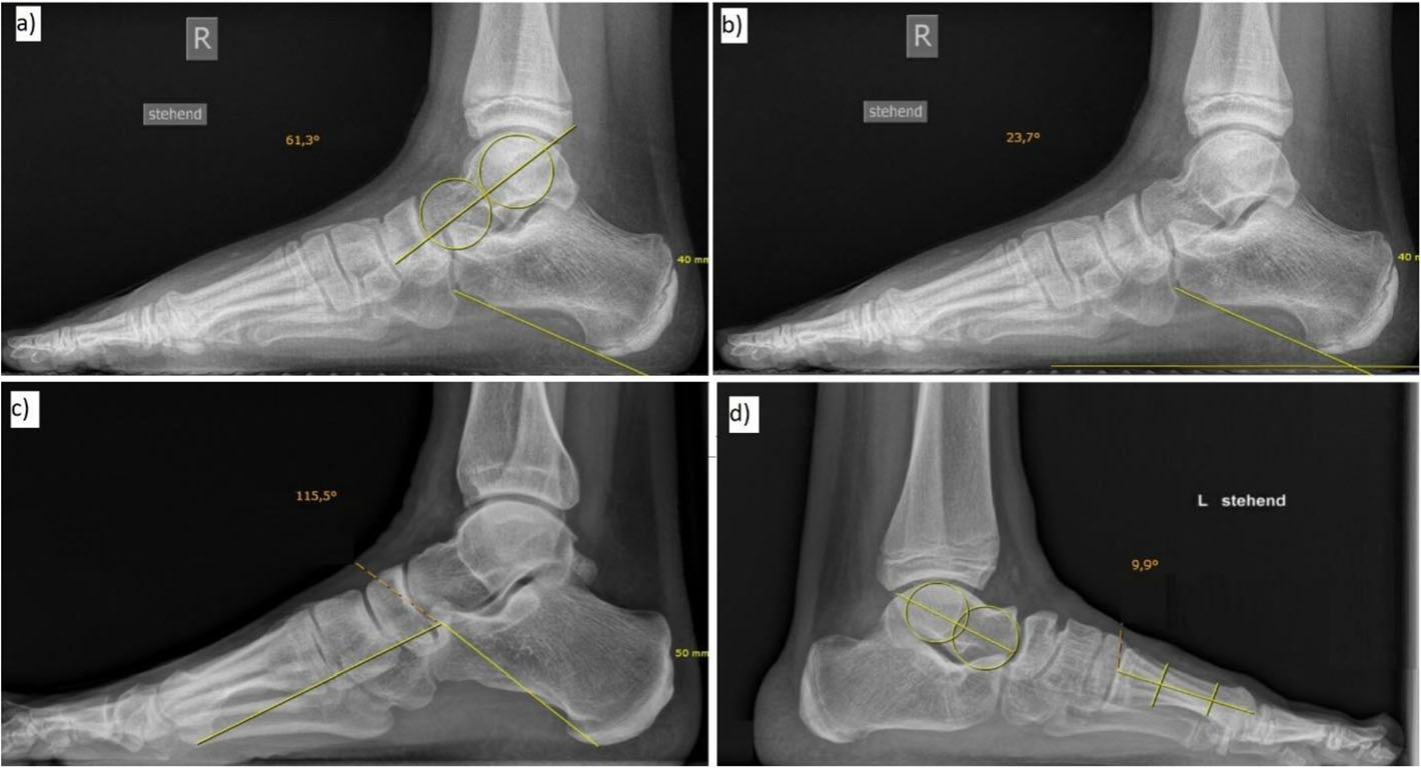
Radiographic parameters evaluated in the lateral view: a Talocalcaneal angle (TC-lat), (b) calcaneal-pitch angle (Calc-P), (c) Costa-Bartani angle (Costa-B), (d) Talo-first-metatarsal angle (TMTI-lat)
